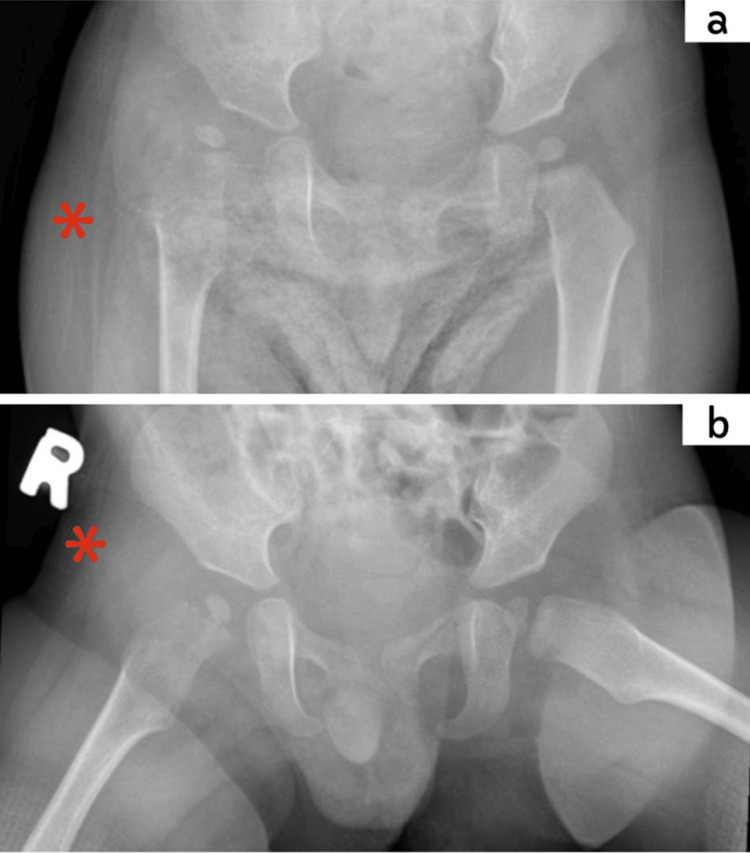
Anteroposterior radiograph of the pelvis with the right hip, marked with red asterisk following surgical arthrotomy, labeled (a) showing subluxation of the right hip joint is evident secondary to capsular and joint destruction. Subsequently, the hip has been reduced and maintained with hip spica application, labeled (b)
